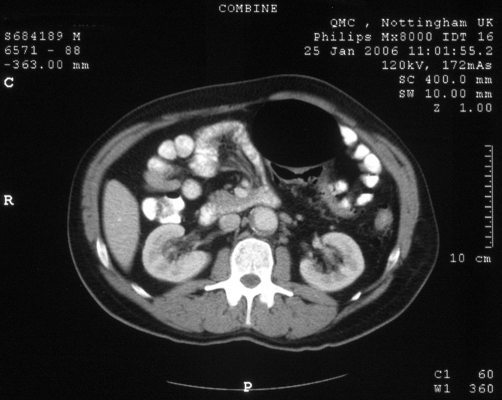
Abdominal computed tomography demonstrating a large gas-filled structure in the left upper abdomen arising from the sigmoid colon.
